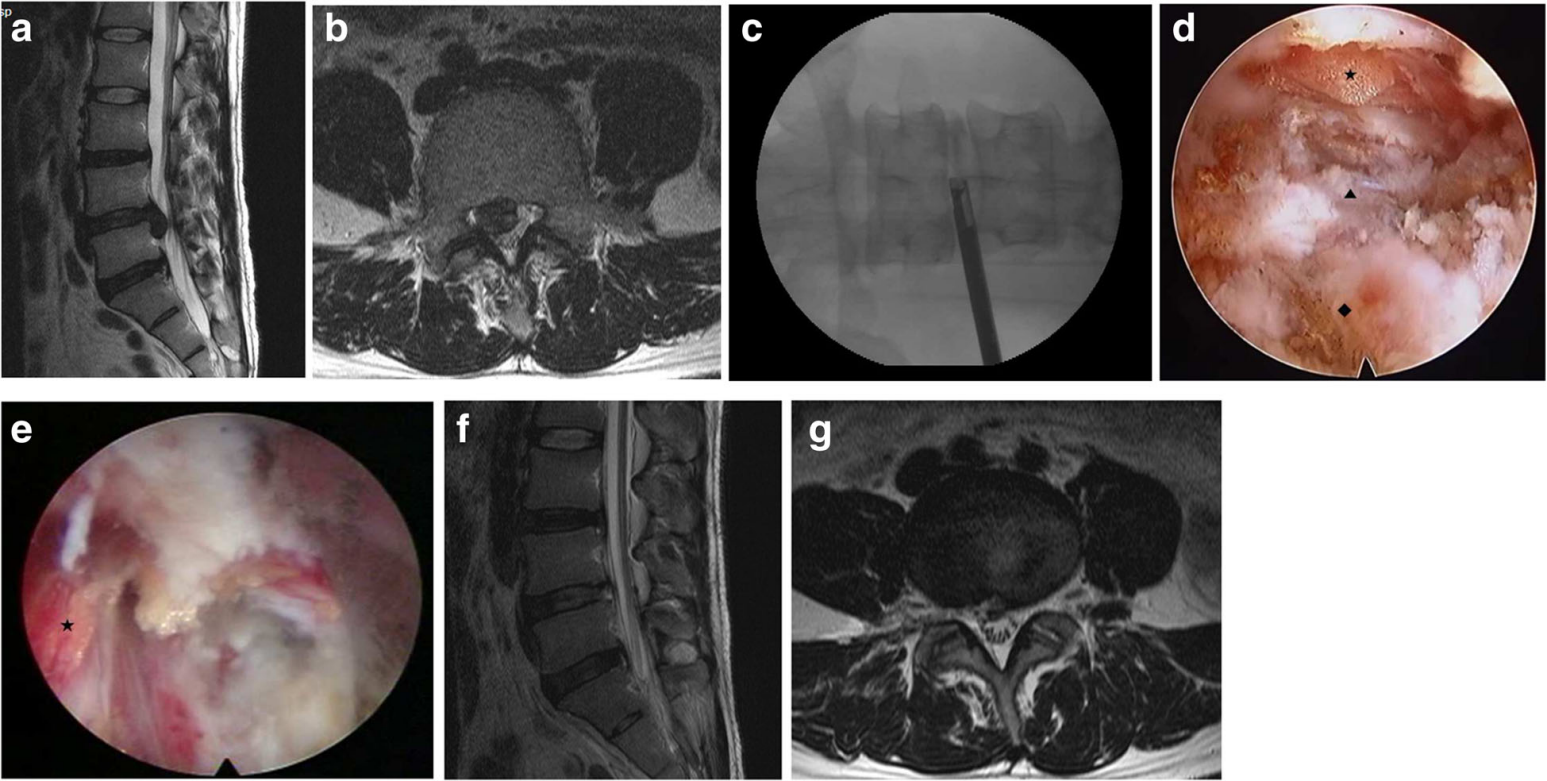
T2-weighted parasagittal magnetic resonance (MR) images showing down-migrated disc herniation at the L4–5 (a and b); after first PELD (c). After removing the nucleus pulposus (◆) and cutting the annulus (▲), the epidural space (★) is found to decompress (d). Pulling out the working cannula, the exiting nerve root (★) is found (e). MR images demonstrating complete removal of herniated disc (f and g)
